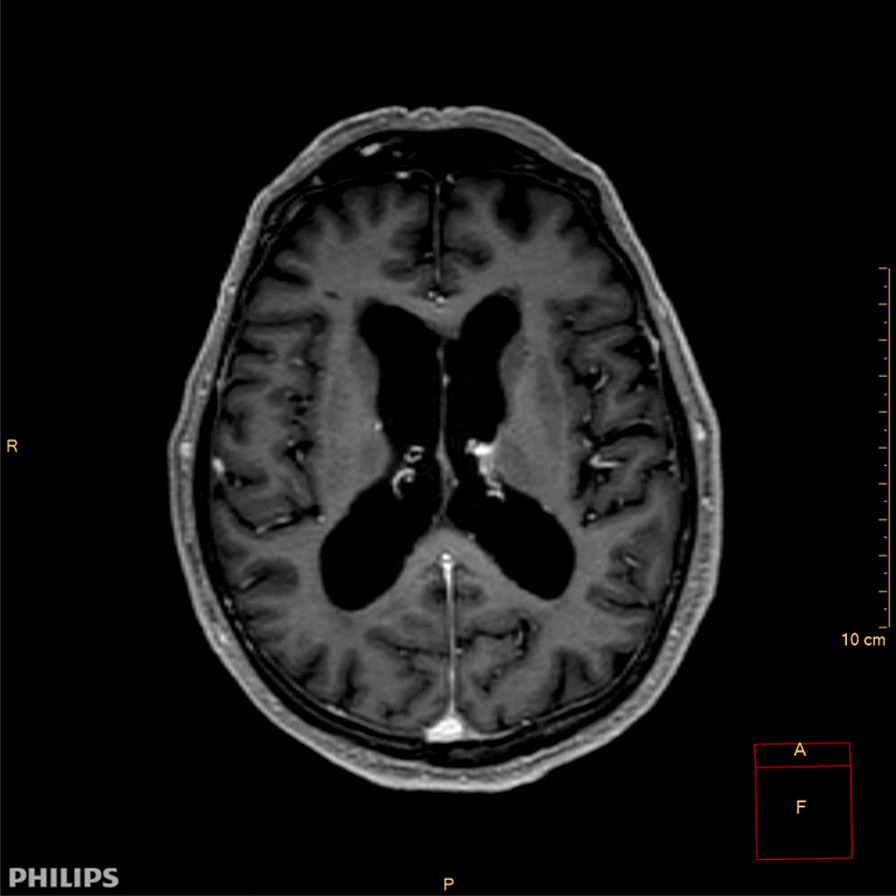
Transverse longitudinal relaxation time (T1) gadolinium enhanced magnetic resonance imaging (MRI) of the brain showing mild central atrophy, otherwise normal. (CM: centimeter; R: right; P: posterior; A: focus; cm: centimeter)
